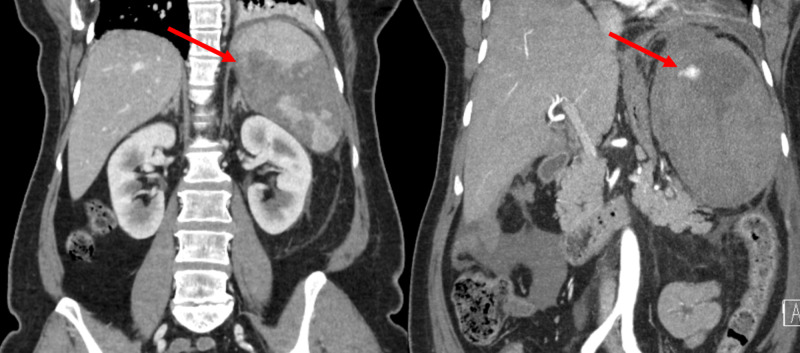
Initial computed tomography (CT) abdominal Imaging Left: Initial CT imaging with the spleen measuring 14.3 cm x 9 cm and an infarct involving > 50% of the parenchyma (red arrow). There is edema surrounding the spleen. Splenic artery and vein are patent. Right: Hospital day 3 contrast-enhanced scan during the arterial phase. Most of the spleen is non-enhancing, compatible with infarction. There is substantial interval worsening of the subcapsular/perisplenic hemorrhage/hematoma formation with the entire process measuring 15 x 10 cm in size now. Active contrast extravasation within the area splenic hemorrhage is demonstrated superiorly (red arrow).
